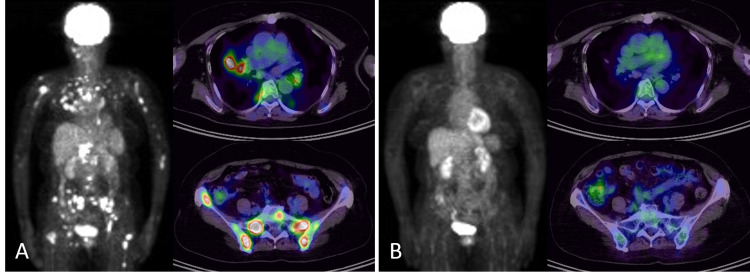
An 18F-fluorodeoxyglucose PET/CT (A) An 18F-fluorodeoxyglucose PET/CT scan showing increased uptake of fluorodeoxyglucose by the mediastinum, nodular lung lesions (SUV: 7.08 (a nodule in the right upper lobe)), abdominal lymph nodes (SUV: 13.38), and foci in multiple bones, including the axial skeleton and pelvis (SUV: 8.08 (the left sacrum)). (B) Four weeks after the start of prednisolone, showing no abnormal uptakes on PET/CT. PET/CT, positron emission tomography/computed tomography; SUV, standardized uptake value
